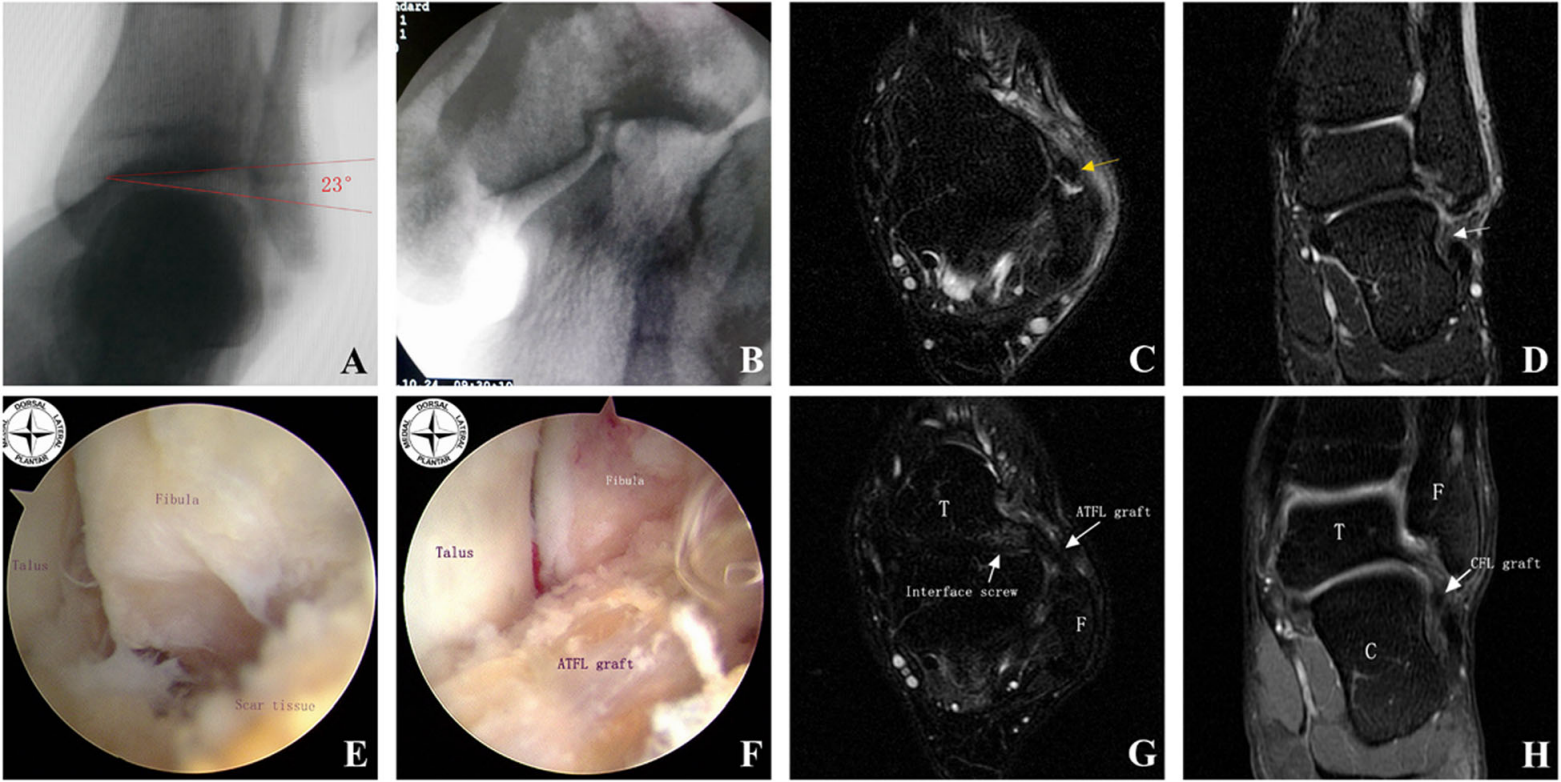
A male patient, age 29, had sprained left ankle joint previously (3 years ago) during practice and subsequently suffered repeated sprains. a and b The results of stress position fluoroscopy indicated that a talar tilt angle range to 23° with the subtalar joint instability. c Sagittal and d coronal views of MRI indicated that the structure of the ATFL was damaged (yellow arrow), and CFL was chaotic (white arrow). e and f Arthroscopic views of the operative site. Severe ligament remnant scarring was found by arthroscopic observation. Suboptimal tendon quality and the semitendinosus tendon transplantation was performed. g Sagittal and h coronal views of MRI indicating good tendon graft for ligament reconstruction at a follow-up 24 months postoperative. Interface screw, ATFL, and CFL were as indicated (white arrow)
